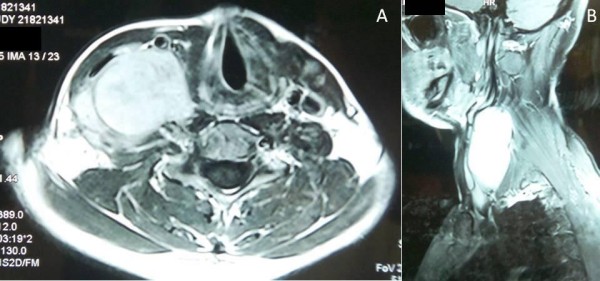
Axial (A) and sagittal (B) T1 MRI scans with contrast showing the mass.
